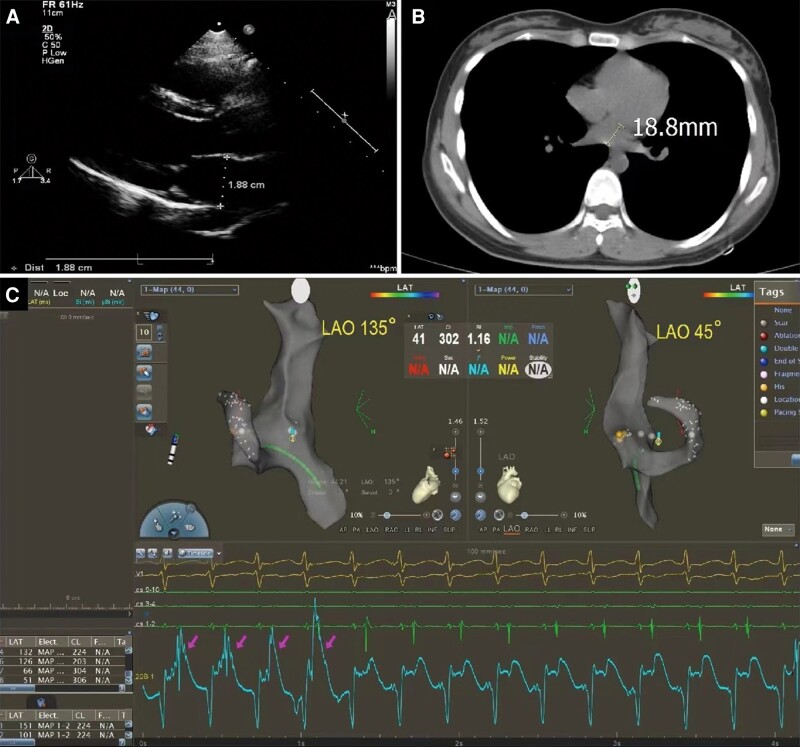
An 8-year-old patient with a small LA underwent fluoroless TSP. The minimum anteroposterior diameter of the left atrium was measured as 18.8 mm using both echocardiography (*A*) and ultra-low-dose CT (*B*). Successful TSPs were guided by EAM and a needle tip unipolar electrogram without the need for fluoroscopic or echocardiographic guidance. High-amplitude atrial potentials with the current of injury (*C*, first four beats, indicated by the pink arrows) could indicate the needle tip location at the muscular limbus of the FO, although in the episode of supraventricular tachycardia. Low-amplitude spiked potentials without the current of injury (*C*, the remaining beats) indicate that the needle tip had reached the central FO. EAM, electroanatomic mapping; FO, foramen ovalis; LA, left atrium; TSP, transseptal puncture.
